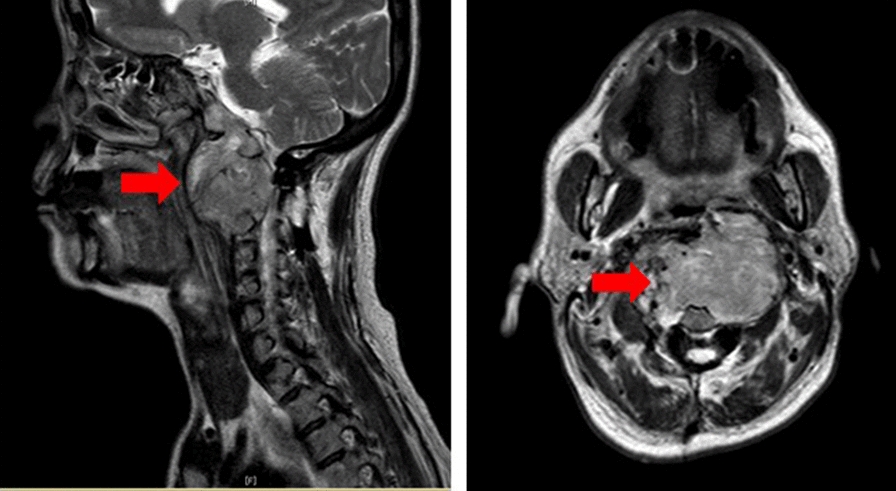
T2-weighted MRI revealed a hyper-intensity signal mass lesion extending from the clivus to the atlas vertebra (red arrow)
